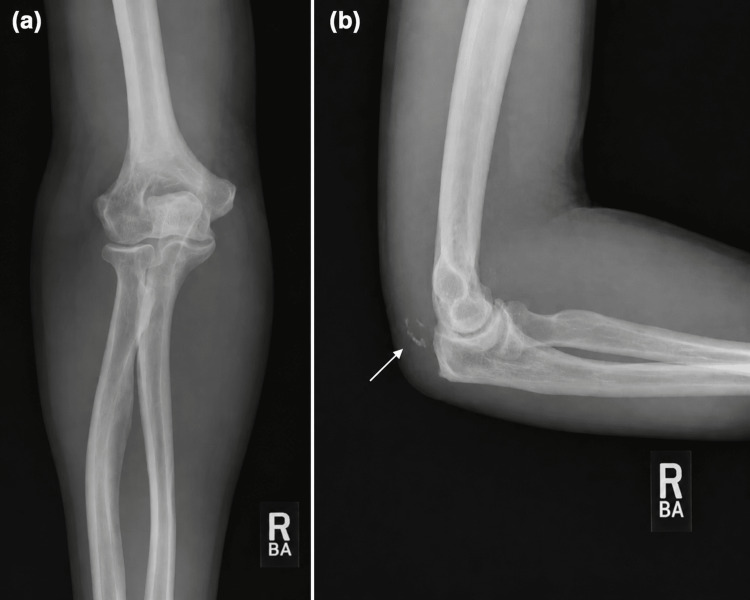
a) Anterior-posterior radiographic view of the right elbow; b) Acute flexion anterior-posterior radiograph with notable avulsion of the olecranon process
